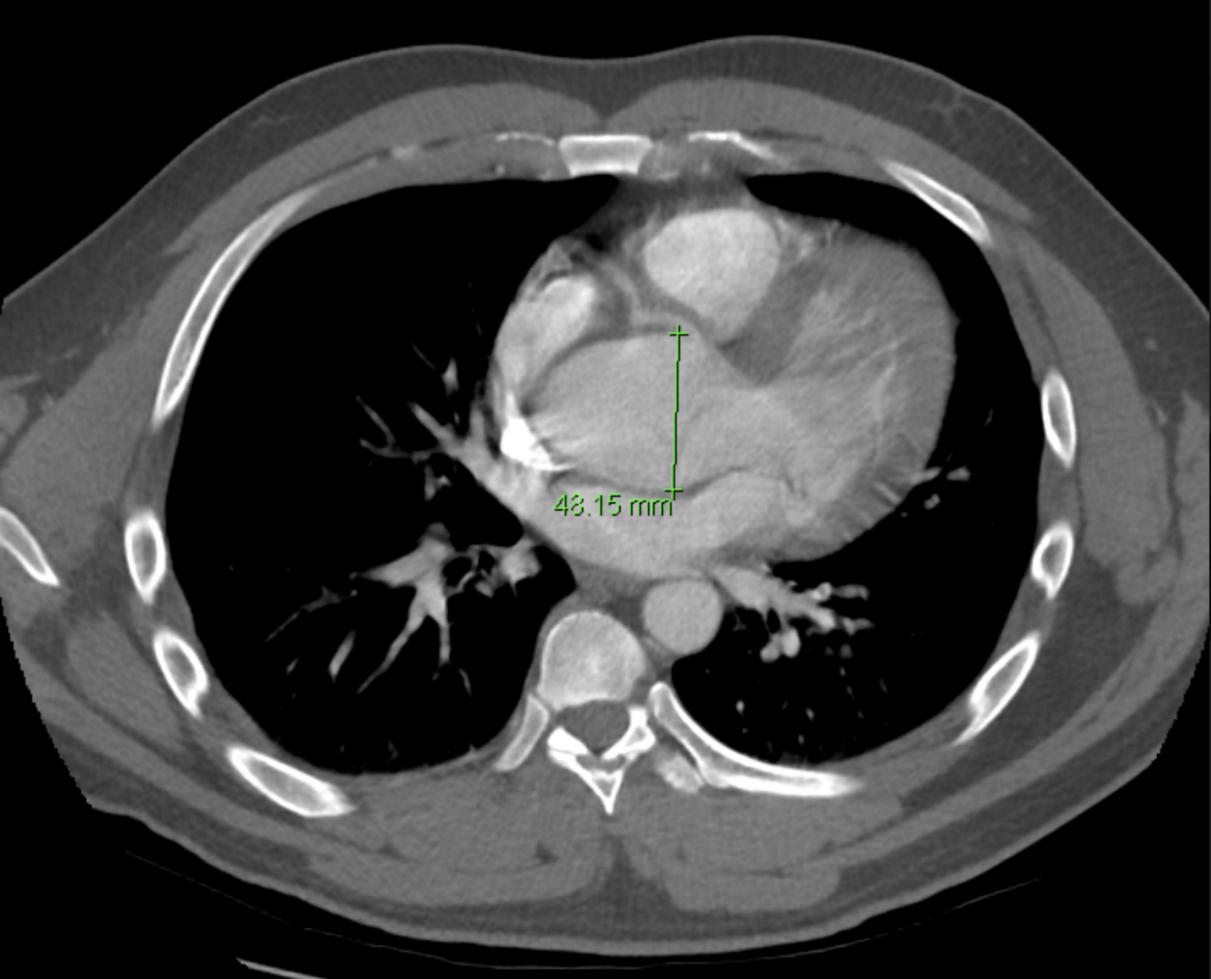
Computerized tomography angiography (CTA) of the chest. Dilatation of the ascending thoracic aorta (from the sinuses of Valsalva to the proximal aortic arch).
